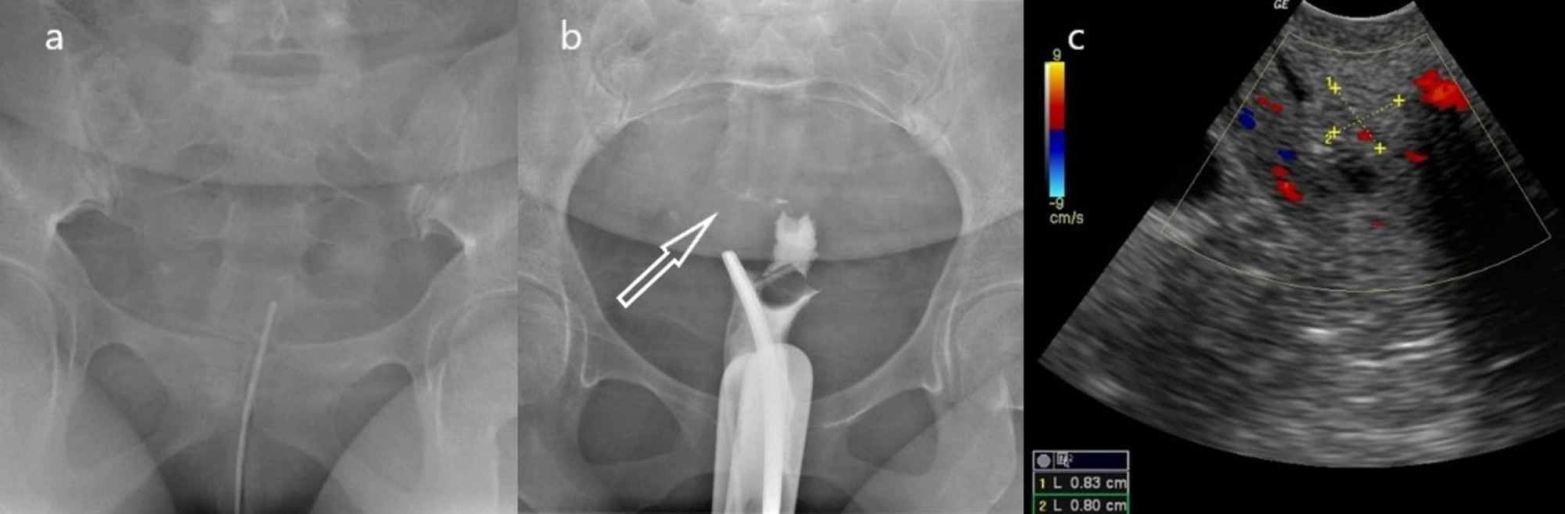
Hysterosalpingography and transvaginal ultrasound images The first image (a) corresponds to the pelvis previous to contrast material injection through the cervical catheter. In the second image (b), total visualization of the cervix is appreciated with a lack of opacification of the endometrial cavity and uterine tubes; the white arrow signals a filiform flow of contrast material in the poorly-distended endometrial cavity. In a transvaginal ultrasound image (c), a round, echogenic, endometrial-dependent structure is observed adjacent to the internal cervical os.
